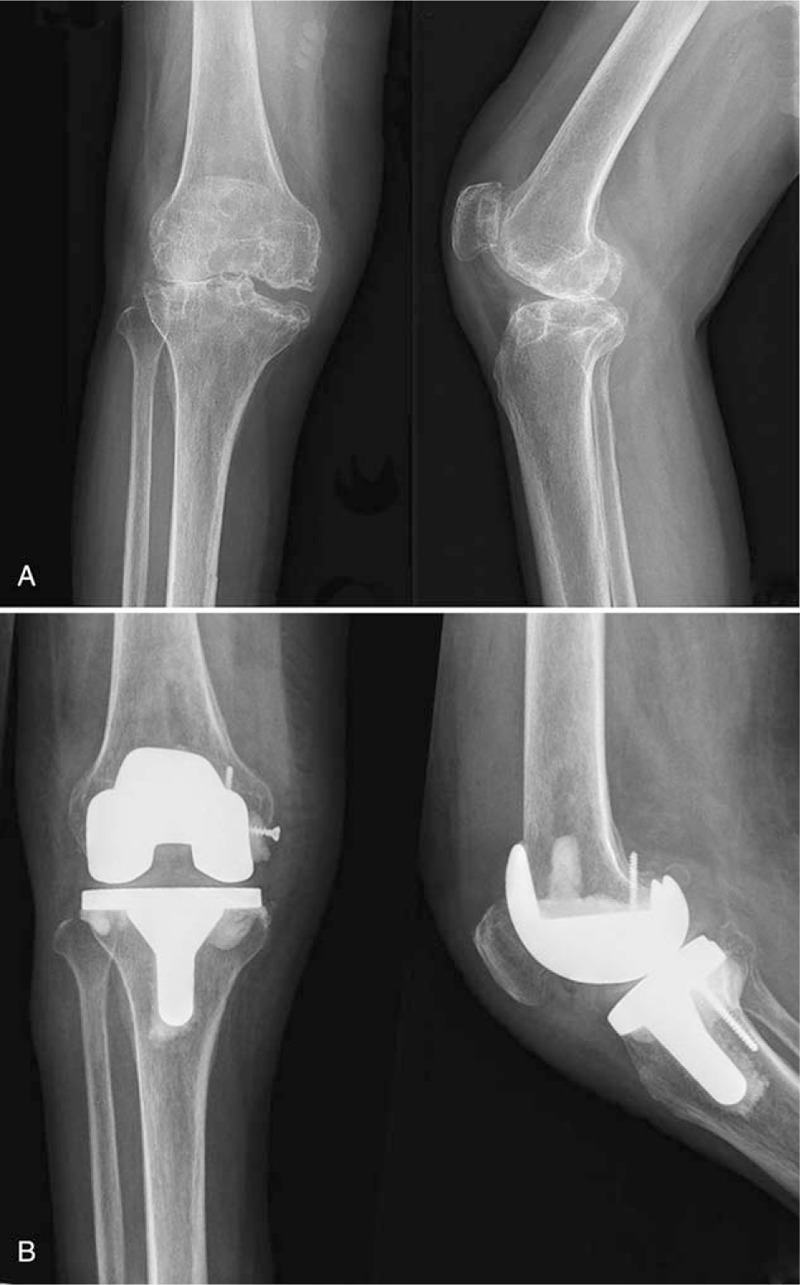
A, X-ray showed severely degenerative and osteophyte formation. B, The prosthesis in good position.
